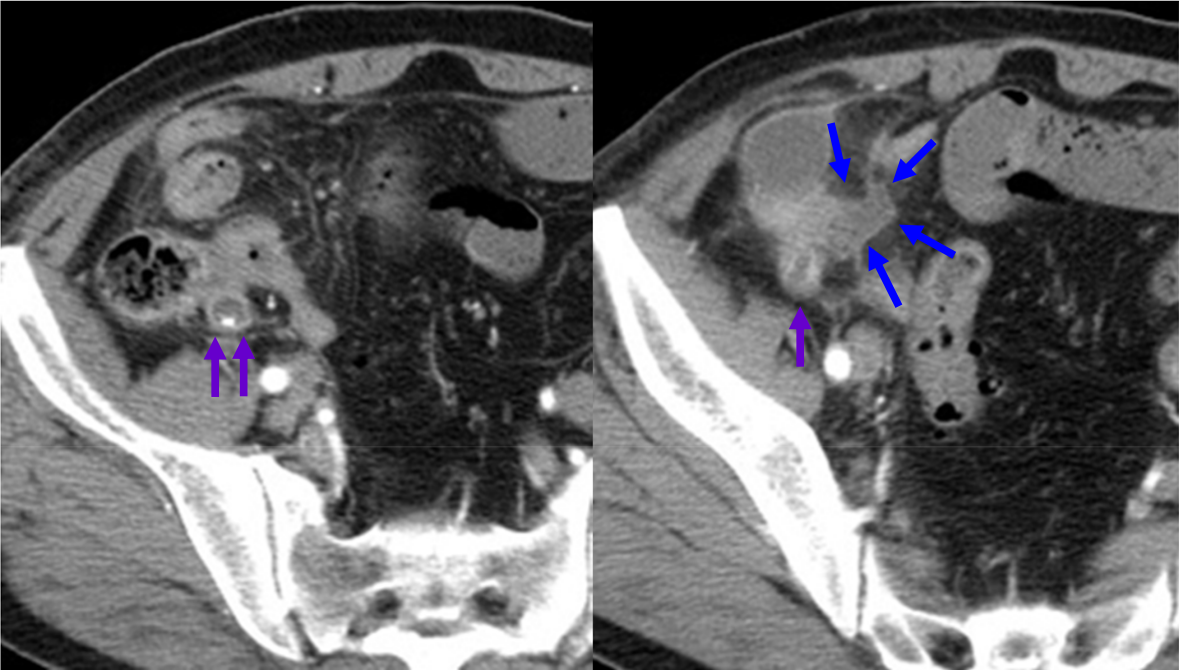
CT findings of acute appendicitis. A swollen appendix (purple arrows) was observed, and fluid collection (blue arrows) near the distal appendix was detected
